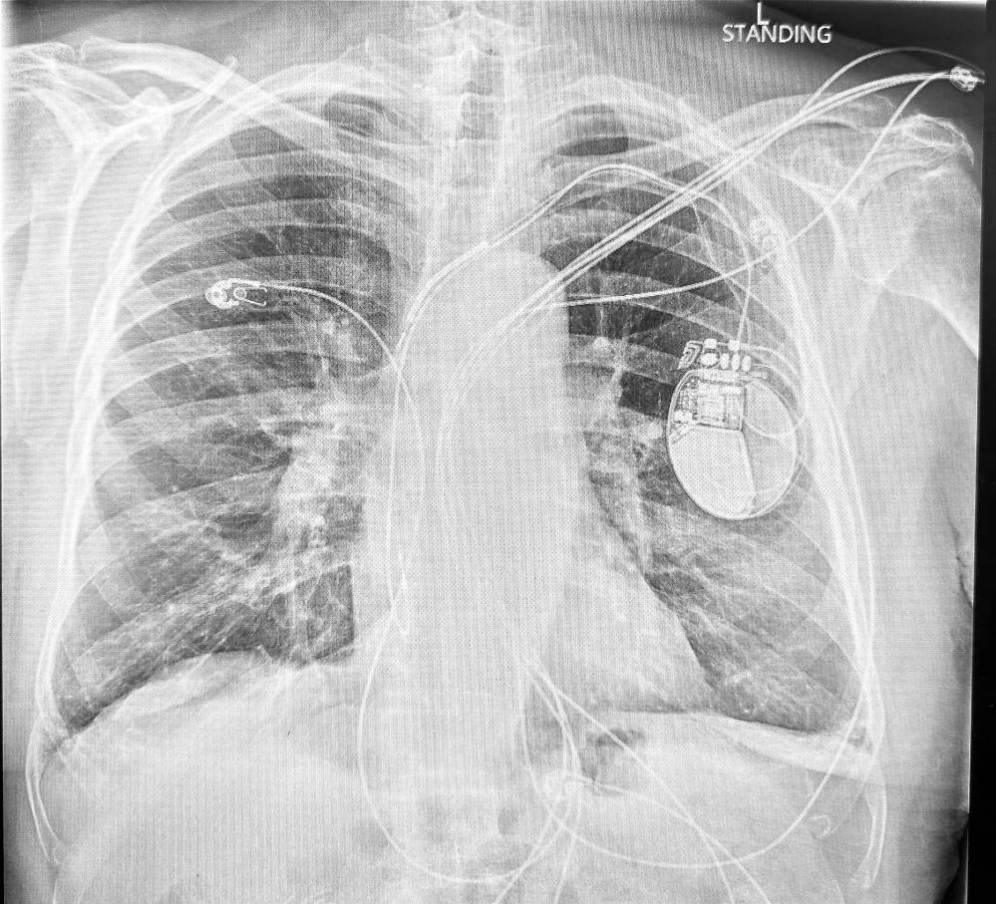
Chest radiograph demonstrating right ventricular lead displacement and coiling into the proximal superior vena cava.
